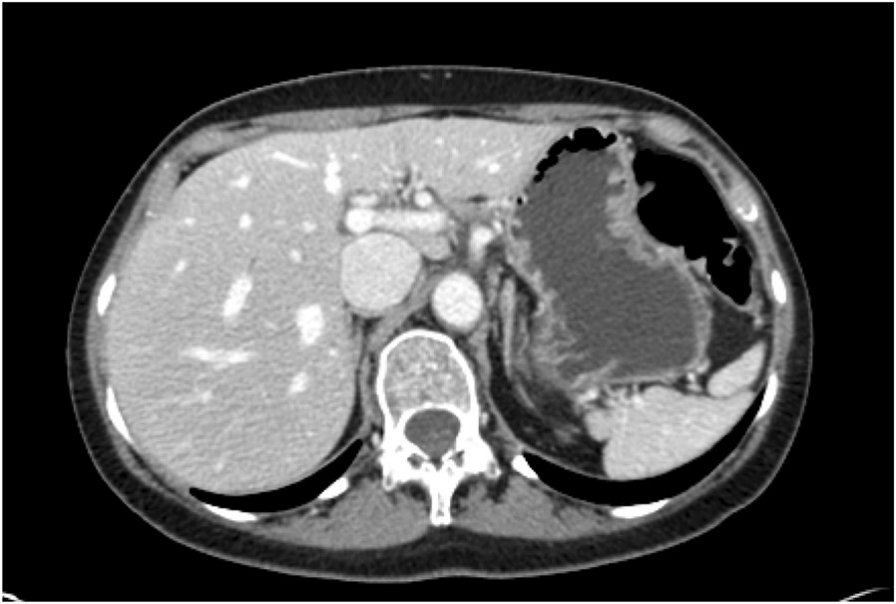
Complete response. In January 2014, CT scan showed a complete response of liver metastases
